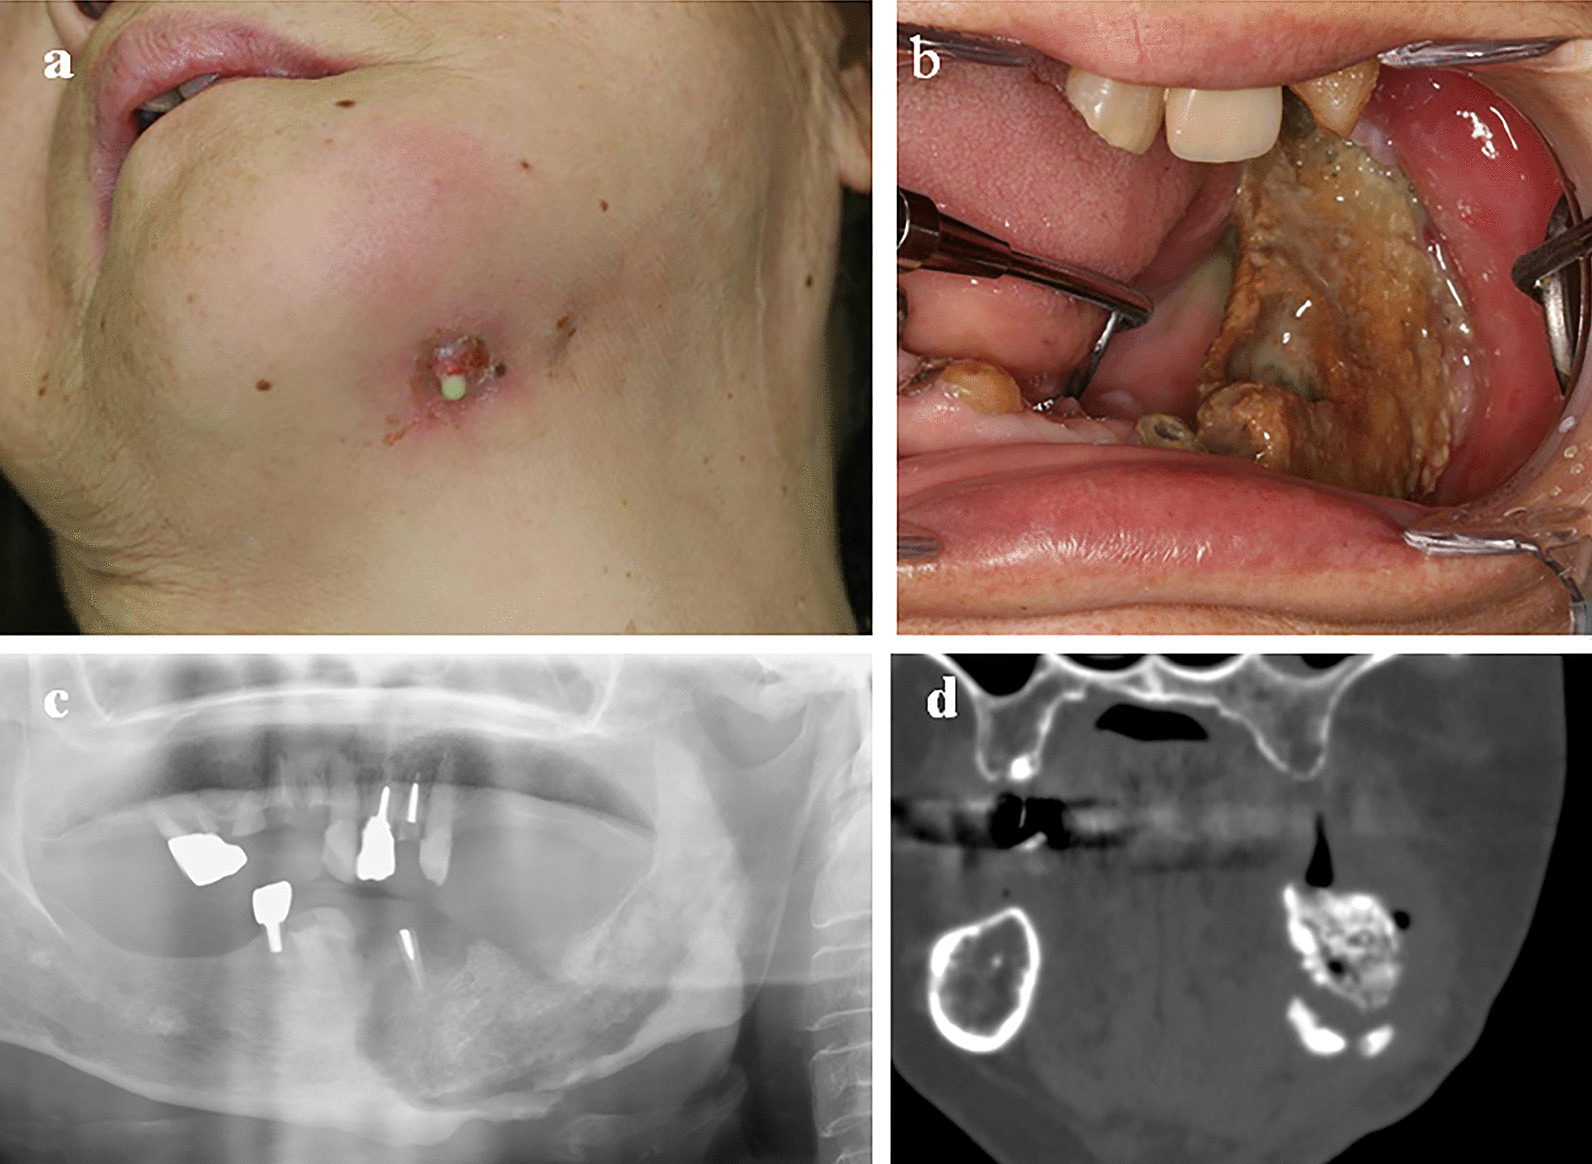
Preoperative appearance and imaging results of our patient. a Lower facial appearance showing a chronic inflammatory reaction with a pustular discharge. b Intraoral view showing necrotic bone and mucosa inflammation on the left half of the mandible. c Panoramic radiograph showing a large sequestrum with a fracture of the left mandible. d Computed tomography scan showing sequestrum and fracture in the left mandibular plane
